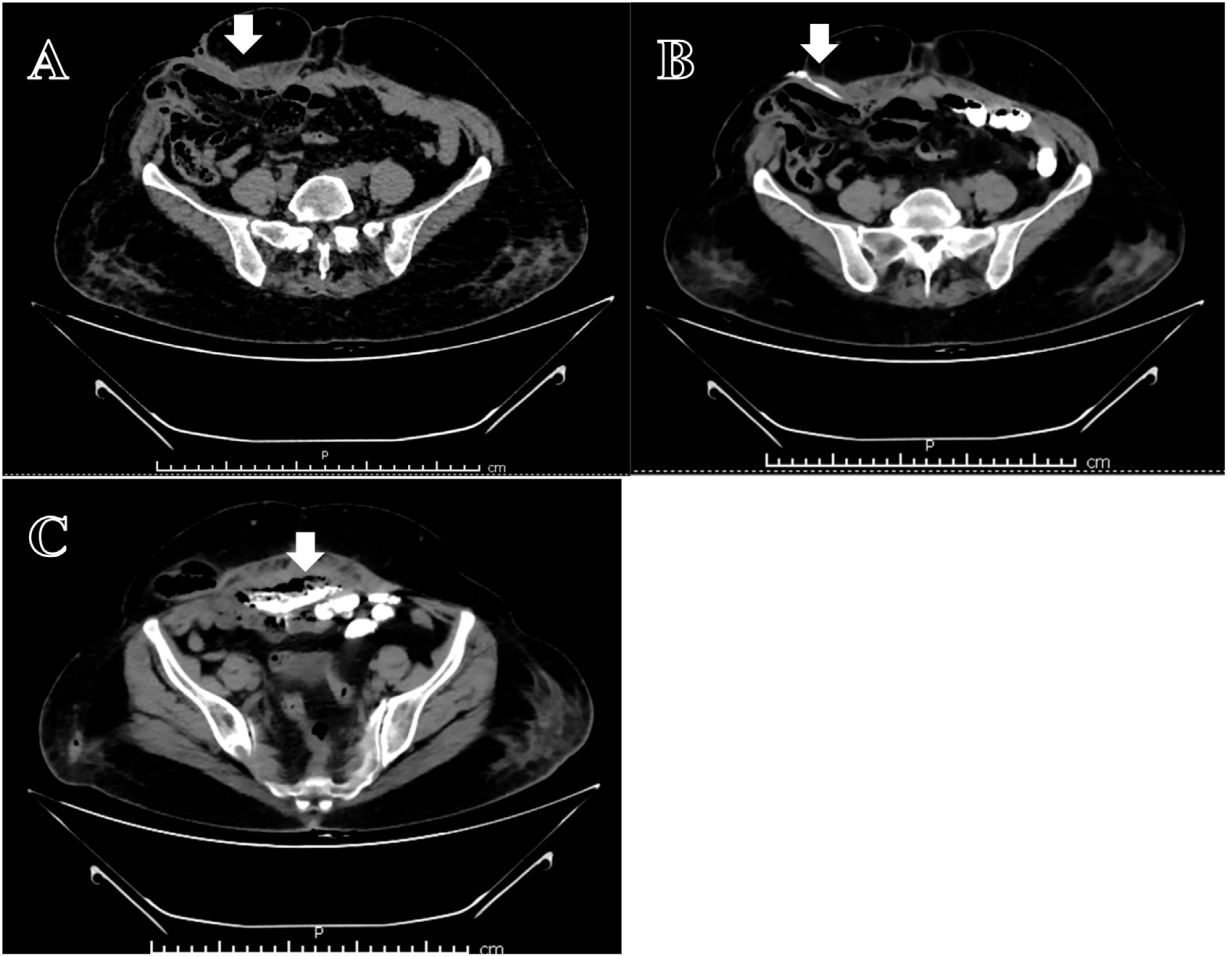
CT Fistulogram: (A) Collection below anterior abdominal wall with external communication (arrow). (B) Contrast fistulogram-showing fistula in right lateral abdominal wall (arrow). (C) Contrast filling in the air containing collection with communication with the bowel (arrow).
